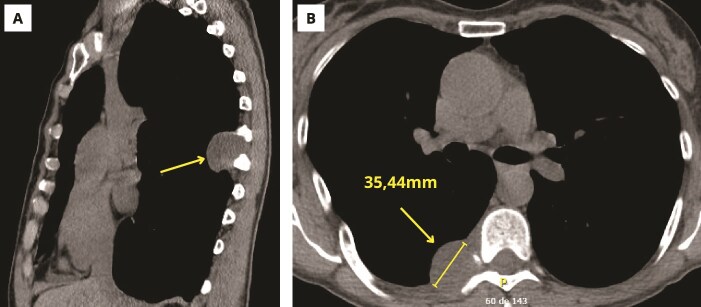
Initial thoracic computed tomography. (A) Sagittal cut. (B) Axial cut.
